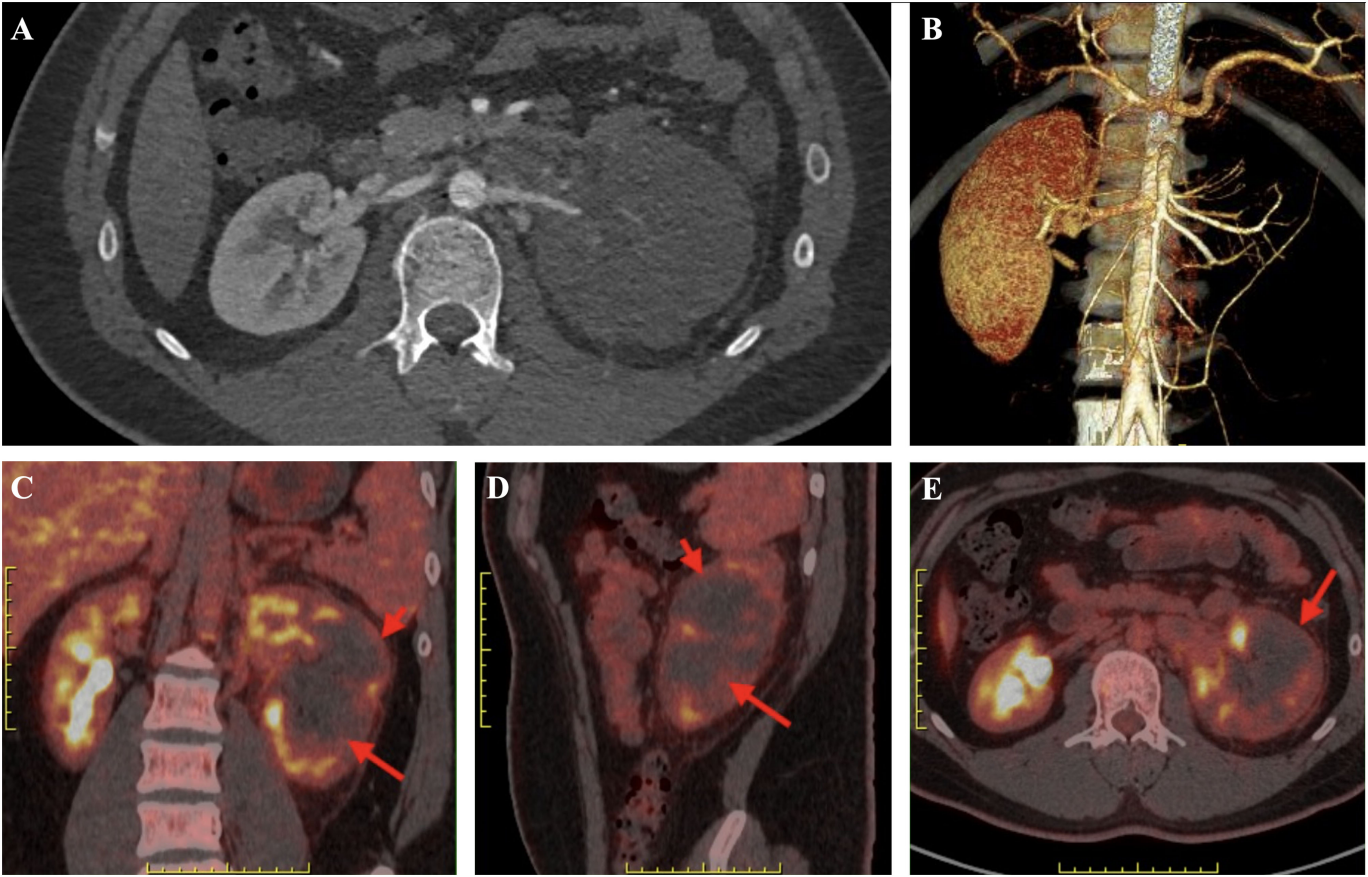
Imaging findings of unilateral left renal infarction with cortical necrosis. (A) Contrast-enhanced abdominal CT (axial) showing a markedly enlarged left kidney with loss of normal corticomedullary enhancement and a peripheral/patchy hypodense appearance consistent with large-area cortical infarction; the right kidney demonstrates preserved perfusion. (B) 3D CT angiographic reconstruction demonstrating markedly reduced arterial supply to the left renal parenchyma (attenuated/absent distal branches), compatible with major arterial occlusion or severe hypoperfusion of the left kidney. (C–E) Fused PET/CT images (coronal C, sagittal D, axial E). Red arrows denote photopenic regions corresponding to infarcted cortex with absent tracer uptake; yellow arrows indicate residual areas of mild tracer uptake likely reflecting reactive inflammation/viable parenchyma.
